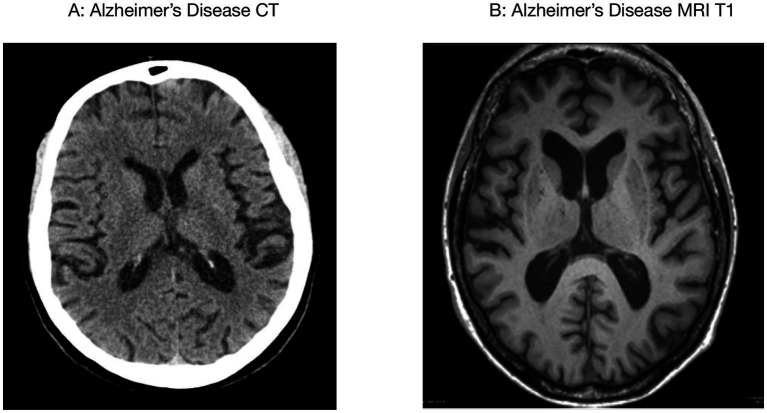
(A) Alzheimer’s disease CT. (B) Alzheimer’s disease MRI T1.
